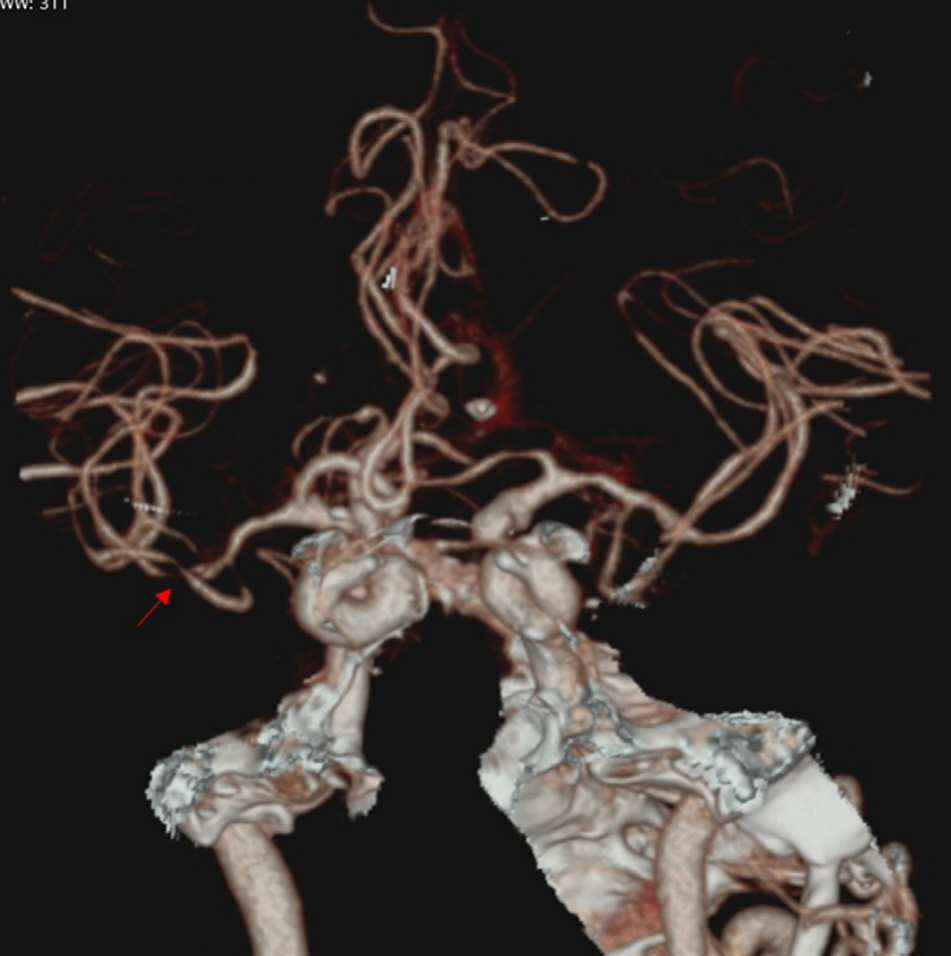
CT Angiography showed arterial patency with stenosis of the right M1-M2 segment (red arrow). (Color version of figure are available online.)
